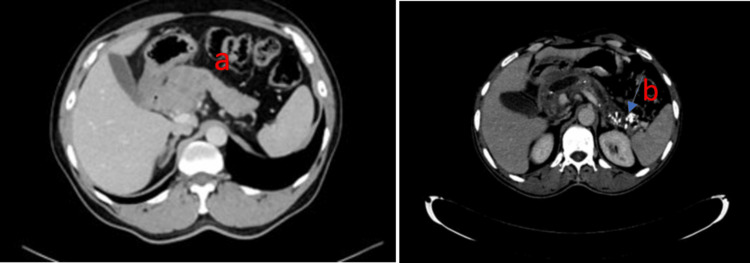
Contrast-enhanced computed tomography (CECT) of the abdomen A- PC patients: a) Carcinoma head of pancreas; B- CP controls: b) Area of CP patients calcification and pancreatic ductal dilatation and pancreatic atrophy PC: Pancreatic cancer; CP: Chronic pancreatitis
